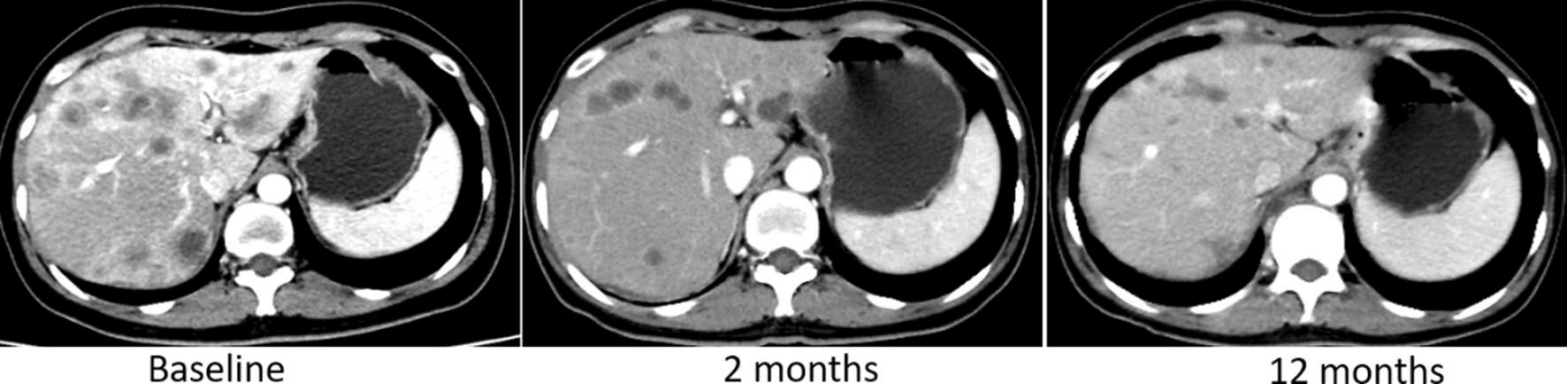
A typical patient’s CT imaging of liver. CT image at baseline showed extensive intra-hepatic lesions. CT image showed the intra-hepatic lesions shrinking after 2 months of HAI regime. Intra-hepatic lesions further shrank after 12 months of HAI regime. This patient was judged as exhibiting a partial response
